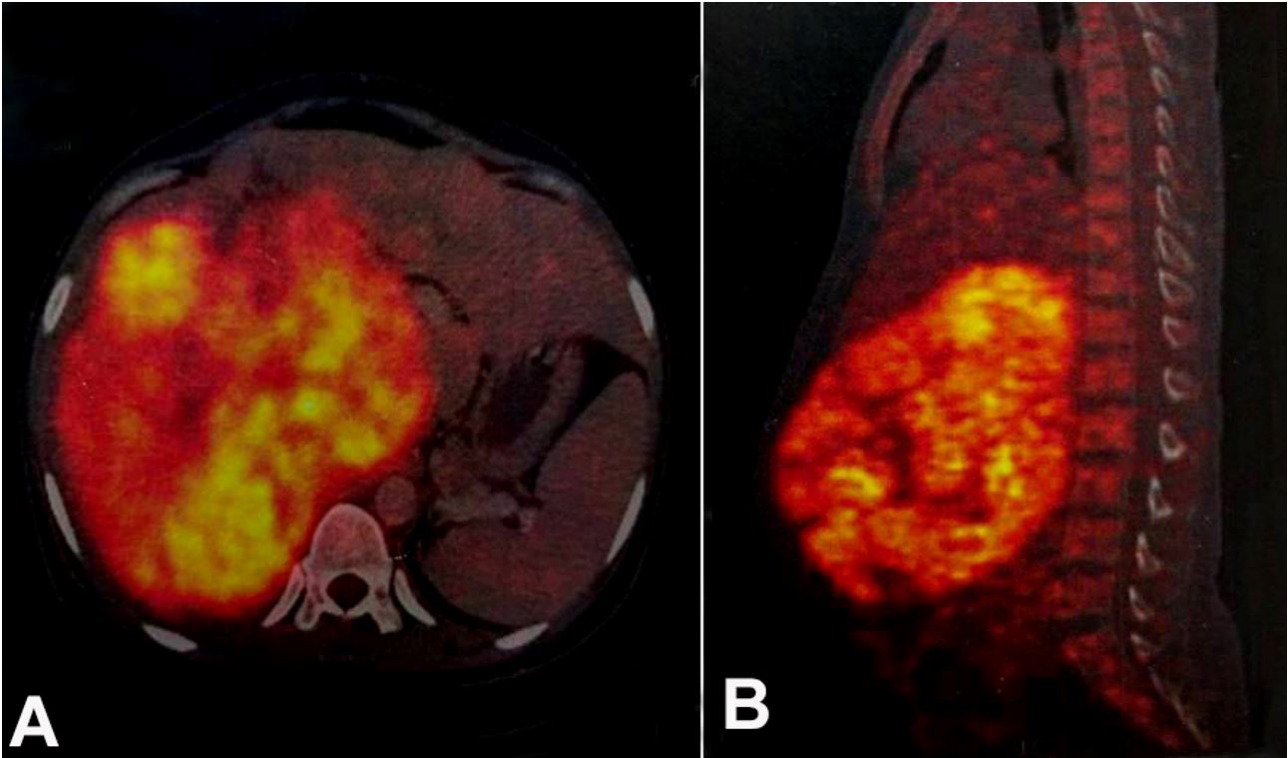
A and B – PET-CT showing large FDG avid heterogeneously enhancing lobulated soft tissue density mass lesion in the right suprarenal region. Right adrenal gland was not separately visualized.
